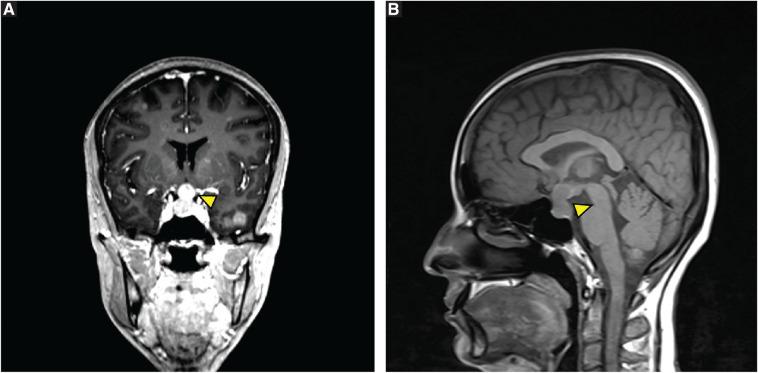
Brain MRI (A) Pituitary enhanced by contrast. (B) Pituitary enlargement. Arrowheads indicate the site of the lesion.
